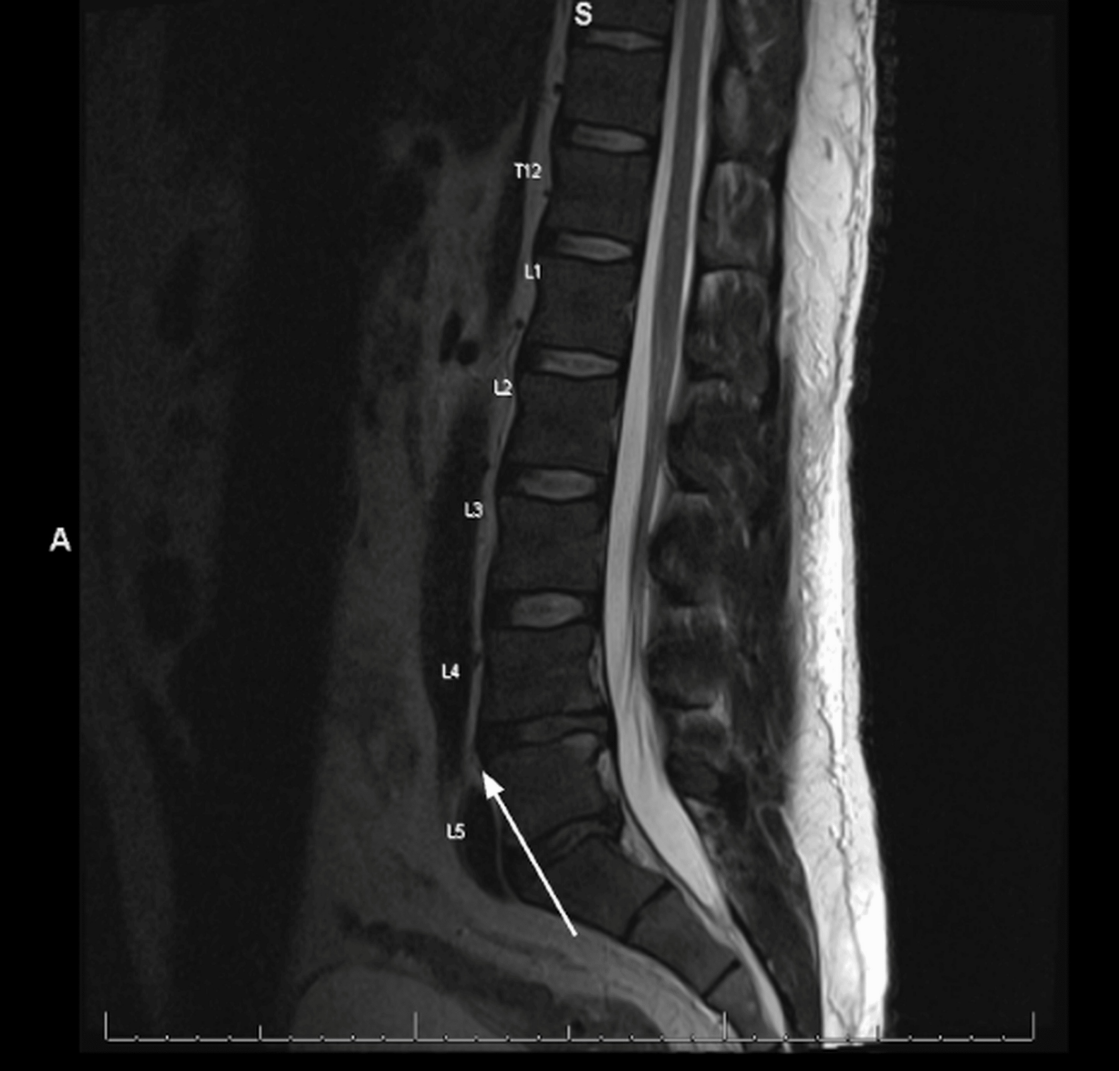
Lumbar spine MRI showing questionable associated epidural enhancement at the L5-S1 level, likely infectious in nature. No abscess or drainable collection was noted.
